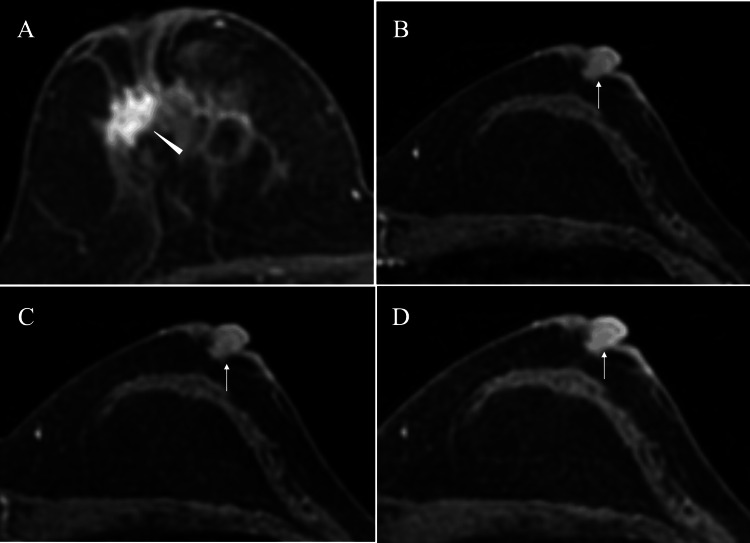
MRI findings at the second operation MRI at the second operation showed non-invasive ductal cancer (arrowhead) in the left breast (A), and no abnormalities in the right nipple-areolar complex (arrow) on fat- suppressed T2-weighted images (B) and on early- (C) and late-phase (D) dynamic study images MRI: magnetic resonance imaging
